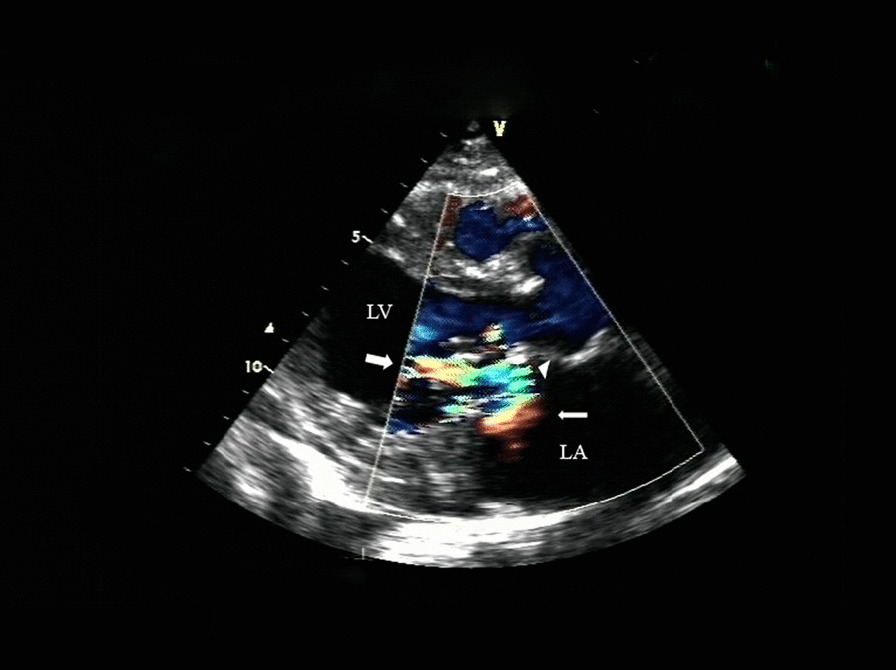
Transthoracic echocardiography showing a jet of mitral regurgitation (large arrow) which immediately collides with the annuloplasty ring (arrowhead), redirecting the jet at a right angle into the central LA (small arrows). LA, left atrium; LV, left ventricle
